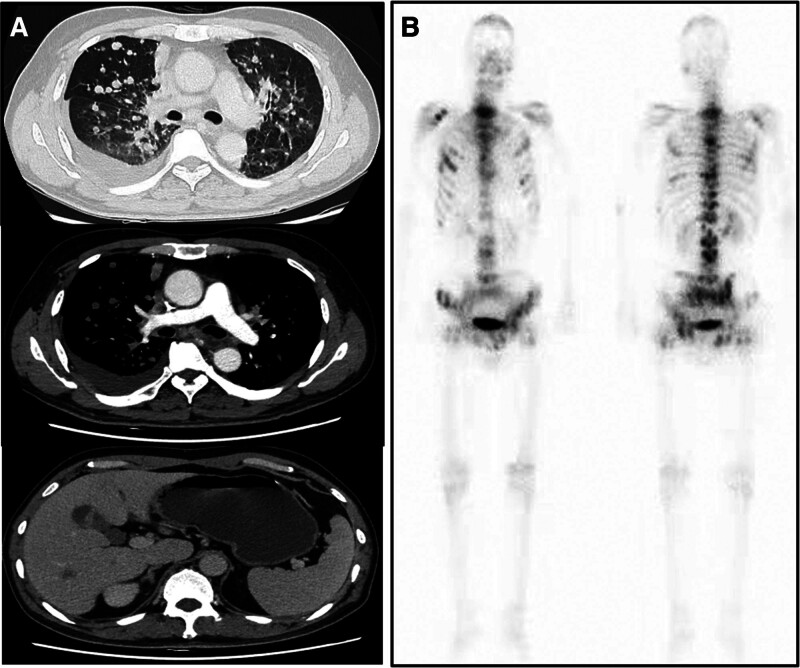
Image examination results. (A) CT showed perihilar lesions in the upper lobe of the right lung with distal obstructive pneumonia, invasion into the adjacent structures of the mediastinum, multiple metastases in the right and left lungs, multiple metastatic lesions in the liver. (B) Bone imaging revealed abnormal increases in the radioactivity of the skull, sternum, cervical, thoracic, lumbar and sacral vertebral bodies, multiple ribs on both sides, scapula, pelvis and lower limbs. CT = computed tomography.
